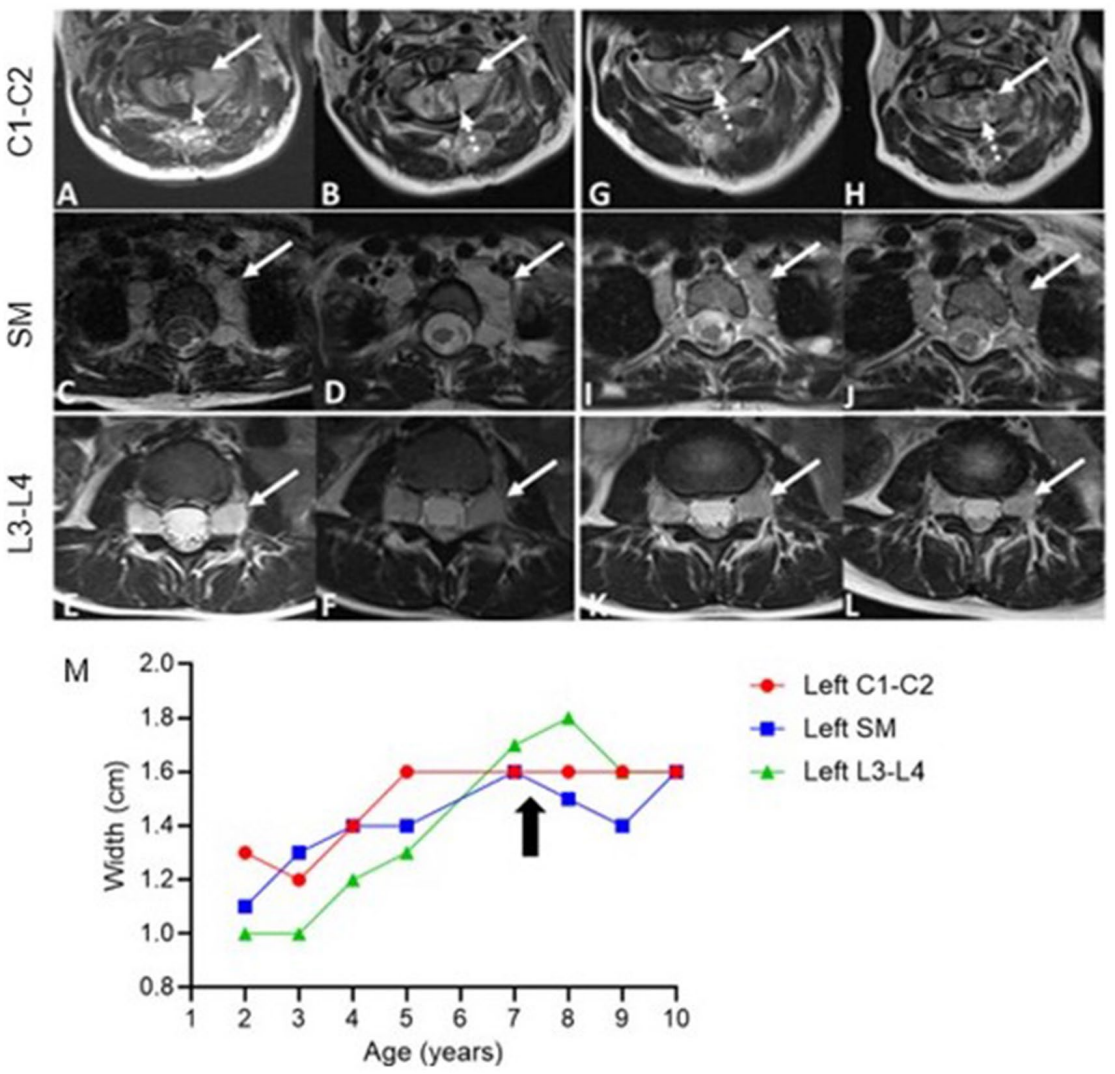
Axial T2-weighted MRI Images pre- and post-treatment. Pre-treatment (A–F) and post-treatment (G–L) images of the cervical spine (C1-C2), superior mediastinum (SM), and lumbar spine (L3-L4), Taken at 14 months (G,I,K) and 28 months (H,J,L) after initiating selumetinib. Cervical spine (C1-C2): Progressive bilateral foraminal nerve root thickening (solid arrow, (A,B)) with post-treatment reduction in intra-spinal thickening (dashed arrow, (G,H)). Superior mediastinum: Bilateral extraforaminal nerve thickening (arrows) shows initial growth before treatment (C,D) and stabilization post-treatment (I,J). Lumbar spine (L3-L4): Persistent bilateral nerve root thickening at the neural foramina (arrows, (E,F)), with no significant change post-treatment (K,L). Measurements of nerve root hypertrophy over time (M). The graph displays the width (cm) of nerve roots at the left cervical spine (C1-C2, red), superior mediastinum (SM, blue), and left lumbar spine (L3-L4, green). The arrow marks the initiation of selumetinib treatment.
